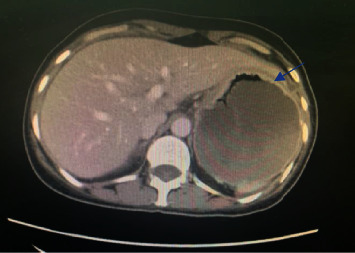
An image of the CT scan of the abdomen and pelvis showing a diffuse gastric wall thickening, about 17 mm (blue arrow).
